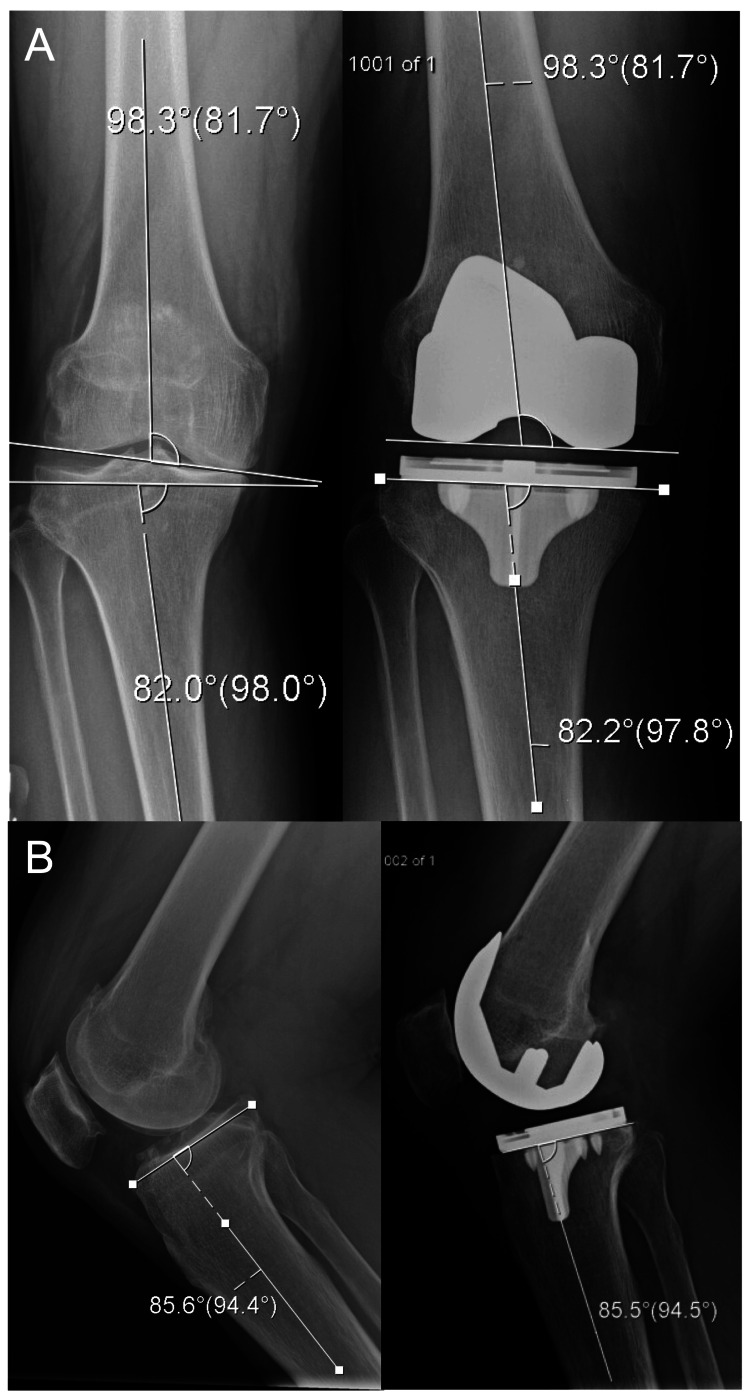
Preoperative and postoperative radiographs (A) AP and (B) lateral radiographs demonstrating a robotically assisted kinematically aligned TKA.
